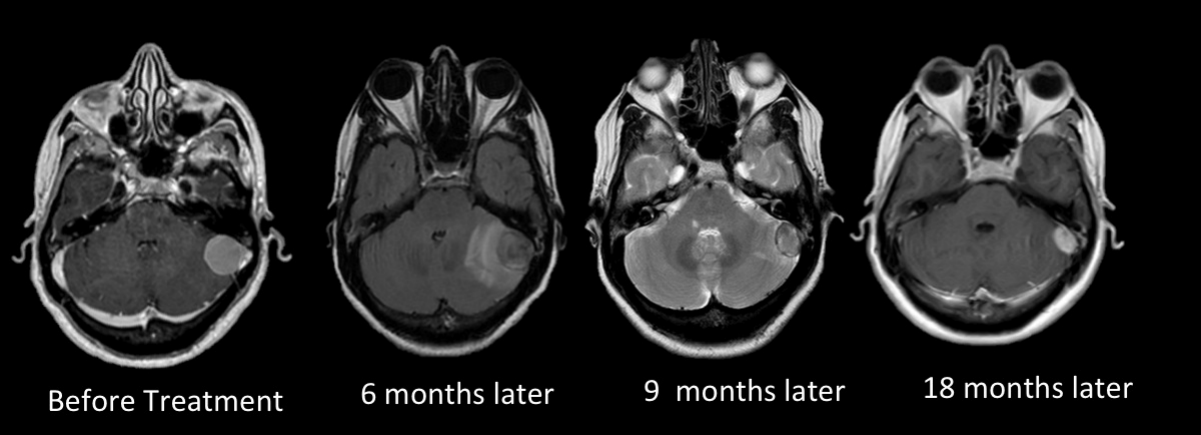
Multisession CyberKnife radiosurgery for a petrosal meningioma in a patient affected by multiple sclerosis. A posterior petrosal meningioma (tumor volume 7.8 mL) in a female patient affected also by multiple sclerosis was treated by multisession radiosurgery (prescribed dose 20 Gy in 3 fractions). Six months after treatment, peritumoral cerebellar hyperintense signal changes appeared on T2 weighed and FLAIR MRI imaging. Symptoms were, however, limited to slight cephalalgia. Low dose corticosteroids were administered to the patient for 3 months. Nine months after treatment, the perilesional signal change disappeared and, at the later imaging confirming control, the tumor showed a remarkable shrinkage.
